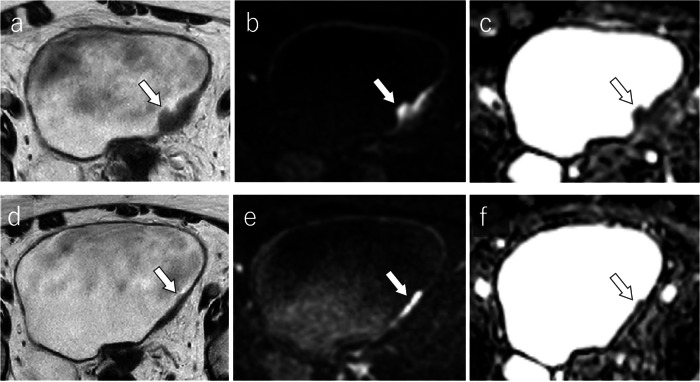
NAC responder: a 76-year-old woman with a 24-mm urothelial carcinoma located in the left posterior bladder wall pre-NAC MRI shows a sessile and broad-based tumor with localized wall thickening and lack of clear disruption of the muscularis layer on T2WI (a) [T2WI VI-RADS score: 3], high-signal intensity on DW-MRI (b) [DW VI-RADS score: 3], and homogeneous low signal intensity on the ADC map with a mean ADC value of 0.579 × 10^−3^ mm^2^/s (c). The tumor is indicated by a white arrow. Post-NAC MRI demonstates reduced tumor volume with slight wall thickening on T2WI (d), linear high signal intensity on DW-MRI (e), and linear low signal intensity on ADC map with a mean ADC value of 0.982 × 10^−3^ mm^2^/s (f). The tumor is indicated by a white arrow. ADC, apparent diffusion coefficient; DW, diffusion-weighted; NAC, neoadjuvant chemotherapy; T2WI, T2-weighted imaging; VI-RADS, vesical imaging-reporting data system
